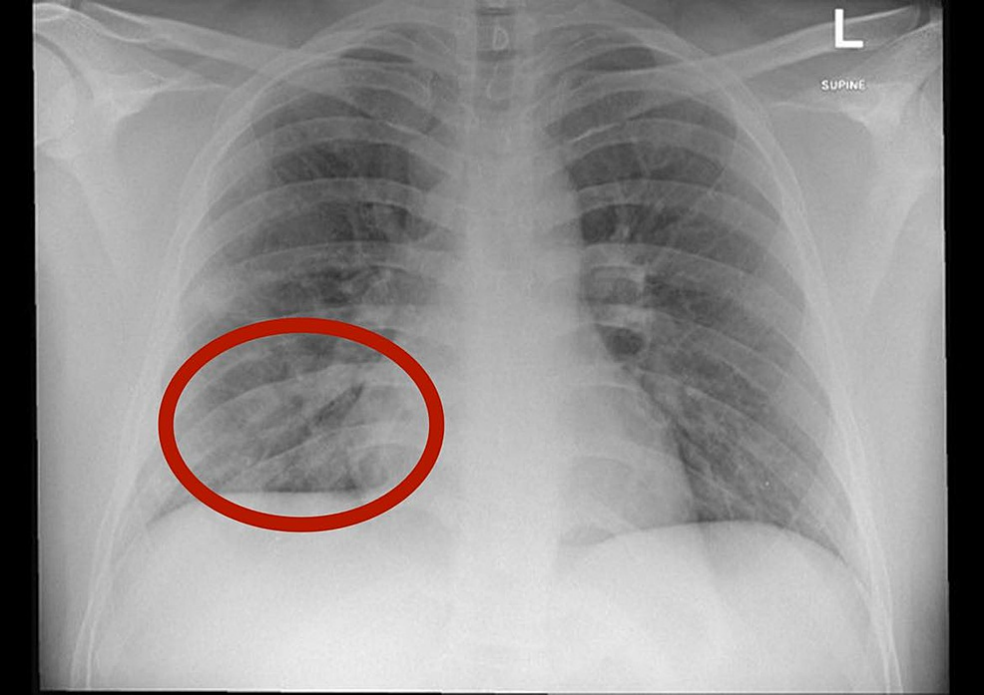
Chest X-ray showing right lobe infiltrates (circle).
